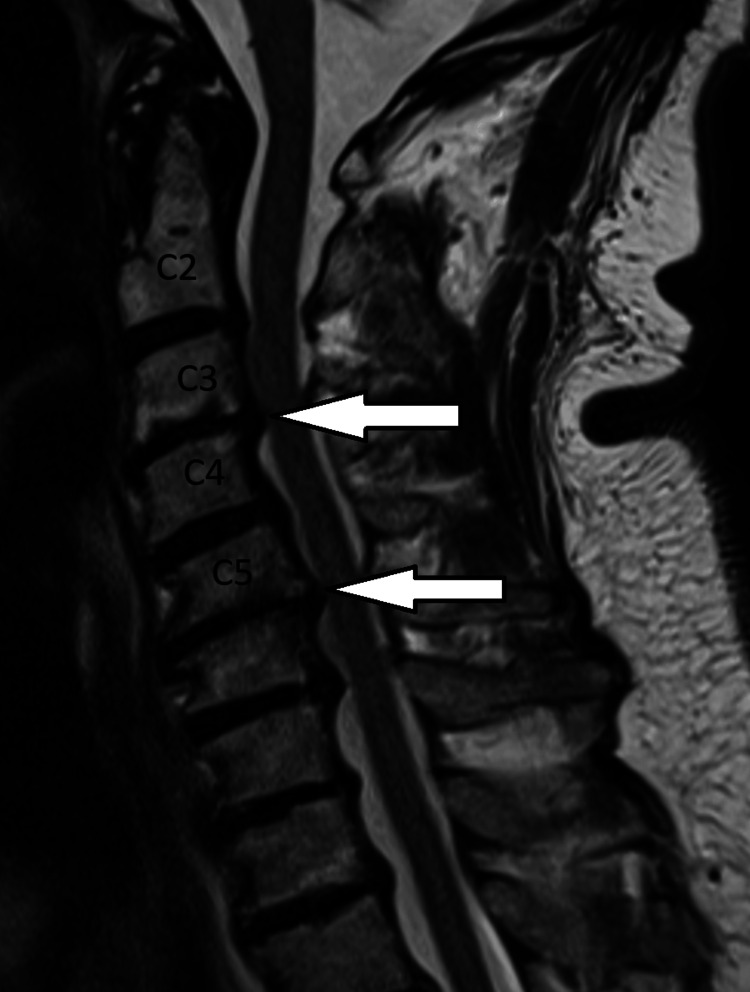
Sagittal T2 sequence MRI spine showing foraminal narrowing, mostly pronounced around C4 and C6 (white arrows). MRI, magnetic resonance imaging.
